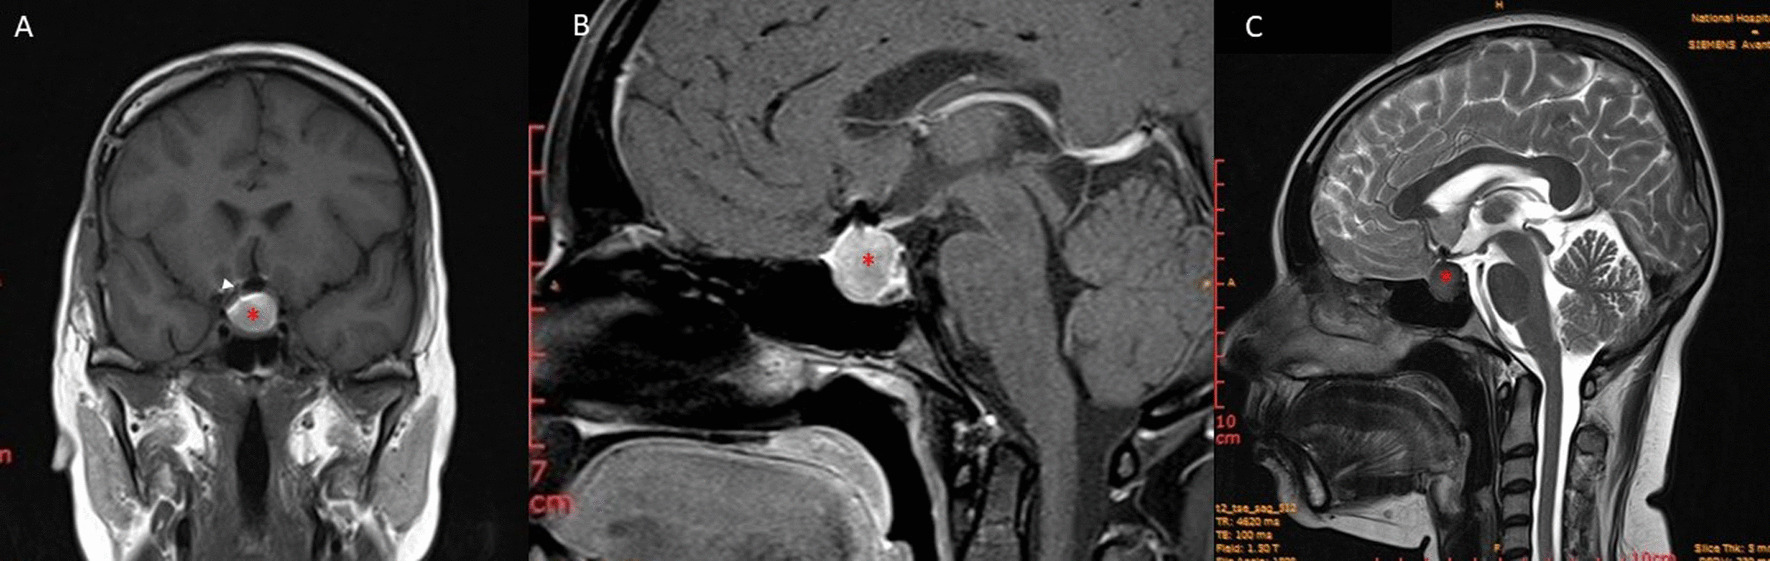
Coronal (A) and sagittal (B) T1 post-contrast and T2 sagittal (C) sections of the magnetic resonance imaging of the pituitary region showing 1.5 cm × 1.7 cm × 1.7 cm cystic mass in the enlarged sella turcica (asterisk). The mass is T1 hyperintense and T2 hypointense. It has suprasellar extension causing optic chiasmal compression (white arrowhead). There is no contrast enhancement
